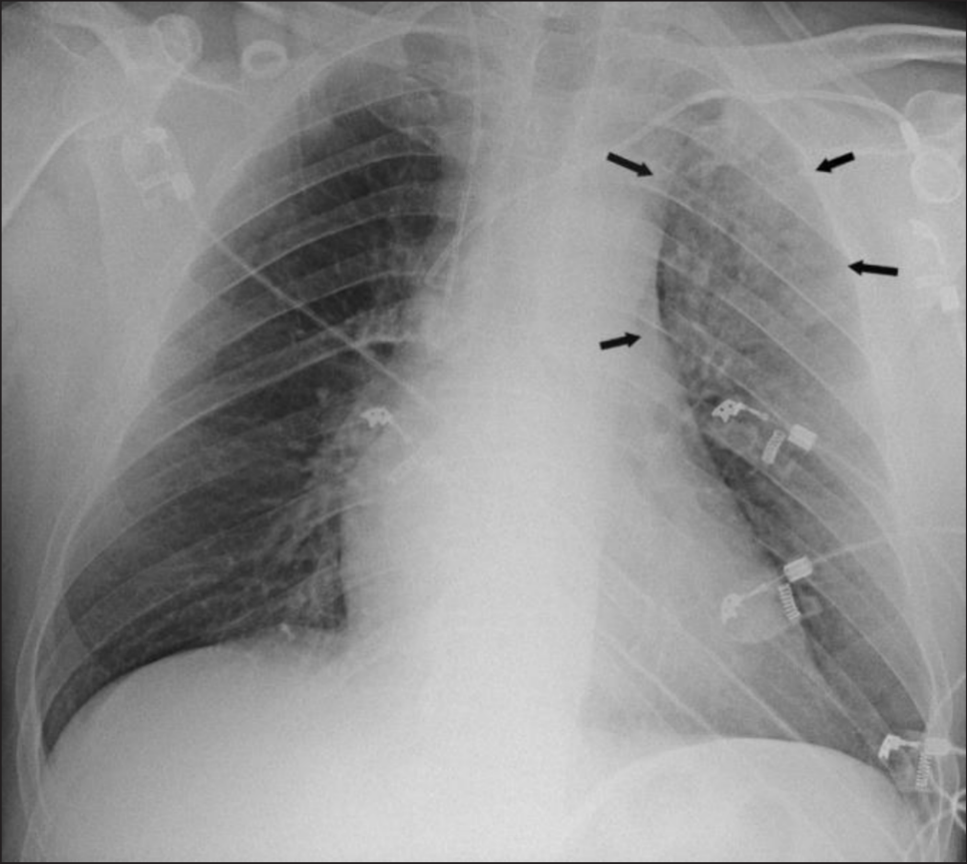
Chest CT angiography (Figure 2) revealed central ground glass opacities in the left upper lobe (black arrowheads), suggestive of pulmonary edema, and a central pulmonary embolism with a saddle clot on main pulmonary artery bifurcation and mostly occlusive thrombi in all lobar and segmental pulmonary arteries except in the left superior lobe arteries (black asterisks). After initial treatment with unfractionated heparin, he was discharged eight days later, with prolonged oral anticoagulant therapy.
